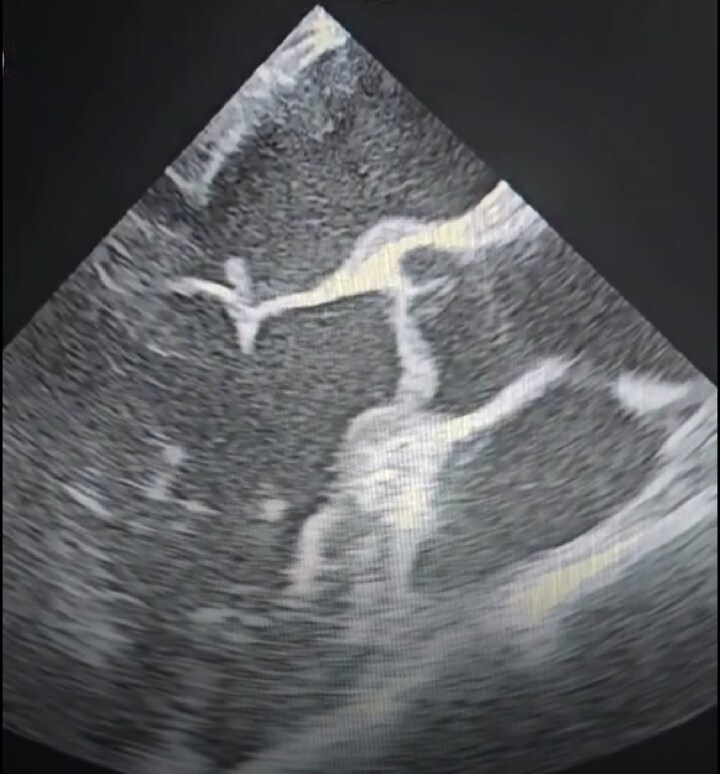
Transoesophageal echocardiographic image: a filamentous, echogenic structure attached to the leaflet coaptation margin in the atrial side of the mitral valve.
